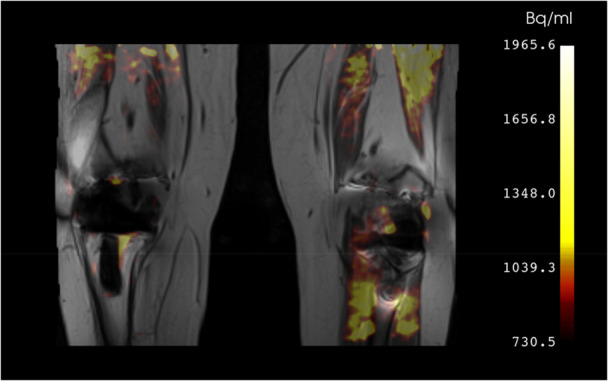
PET/MRI of a patient with bilateral total knee arthroplasty showing uptake of [18 F]FEPPA.
